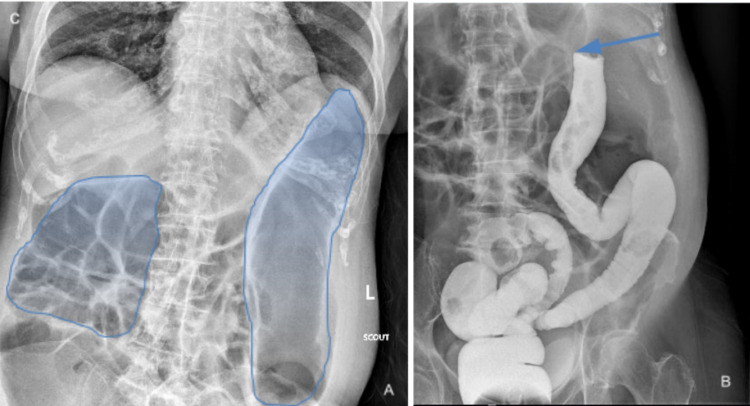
X-ray of the large intestine showing dilated loops of bowel with loss of haustra in descending colon as highlighted in image (A). Gastrograffin enema in image (B) shows splenic flexure obstruction (blue arrow).
